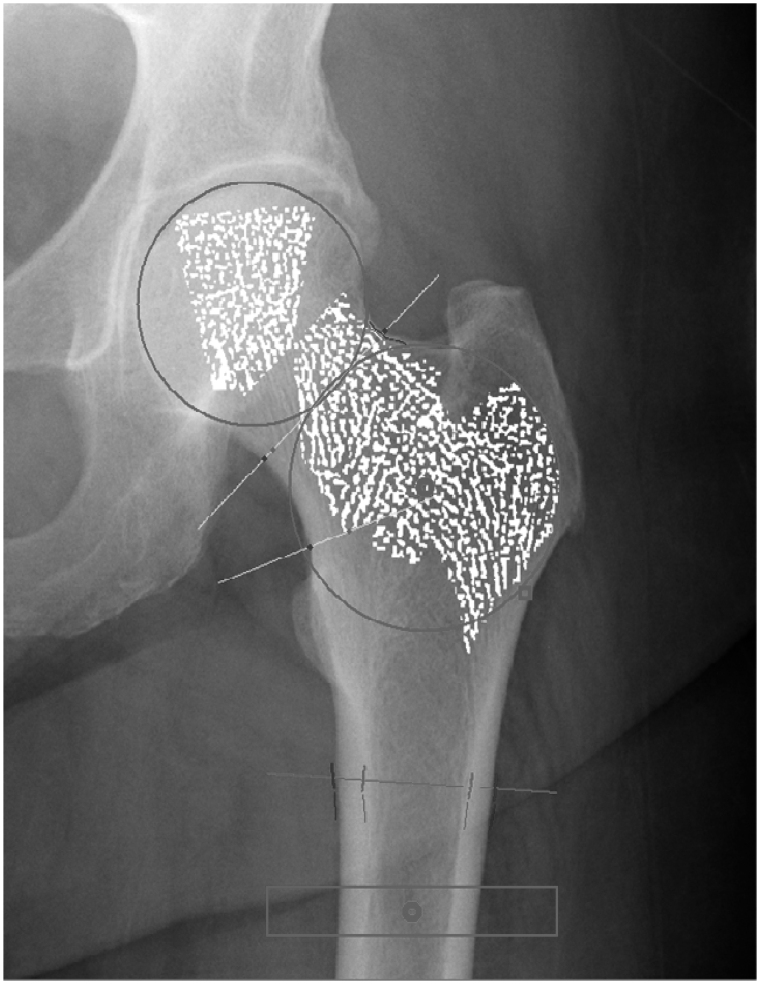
Example Hip X-ray image. Example X-ray image from one of the cases enrolled in the study. Cortical thickness measurements are shown by overlaying red-blue markers placed at several locations along the cortical bone boundary. The highlighted structures on the femoral head, neck and trochanteric regions correspond to the underlying projected bone structures (trabeculae) extracted for further analysis and measurements.
